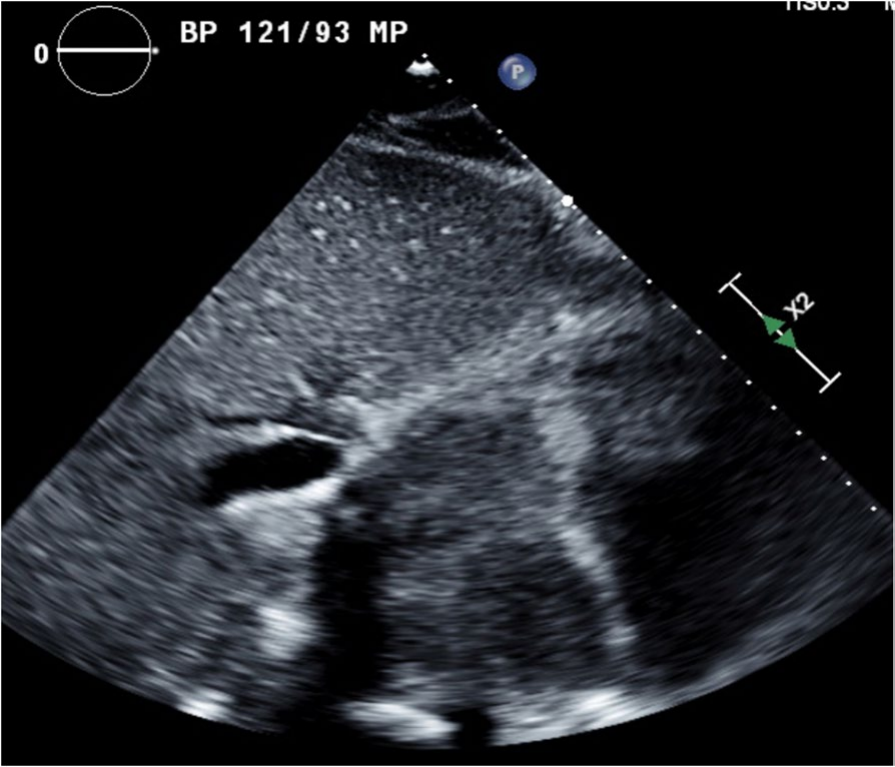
Echocardiogram with subcostal four-chamber view showing large atrial mass occupying both left atrium and right atrium, obliterating the interatrial septum
